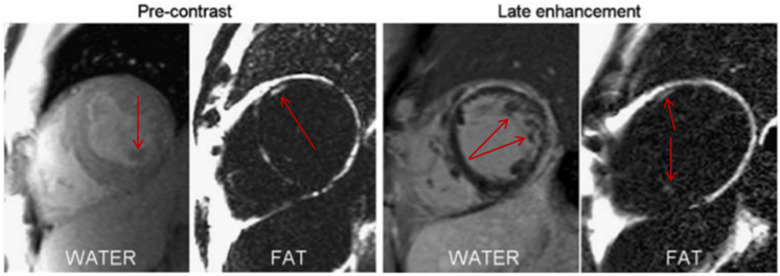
Fibrofatty infiltrates highlighted in fat-water separated MRI pre-contrast (left) and late enhancement (right) in a patient with non-ischemic cardiomyopathy. Reproduced from Kellman et al. [46] under the Creative Commons Attribution Noncommercial License.
